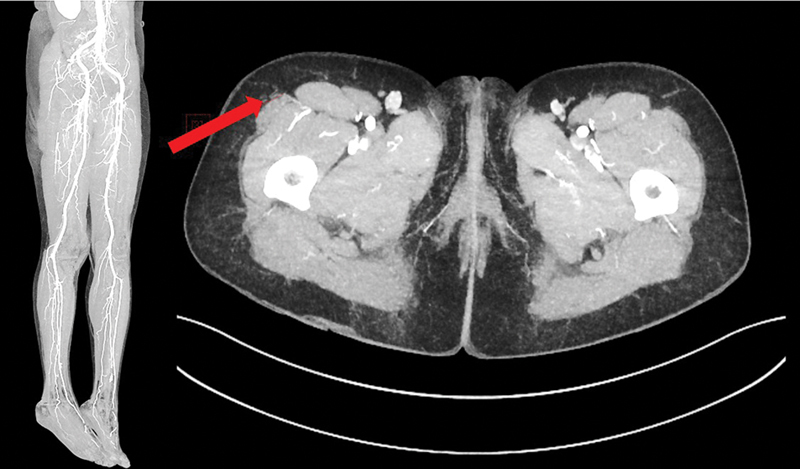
Preoperative computed tomography angiography shows patent lower extremity arteries (bilateral) and an adequate perforator of the descending branch of the lateral femoral circumflex artery of the right leg (red arrow).
